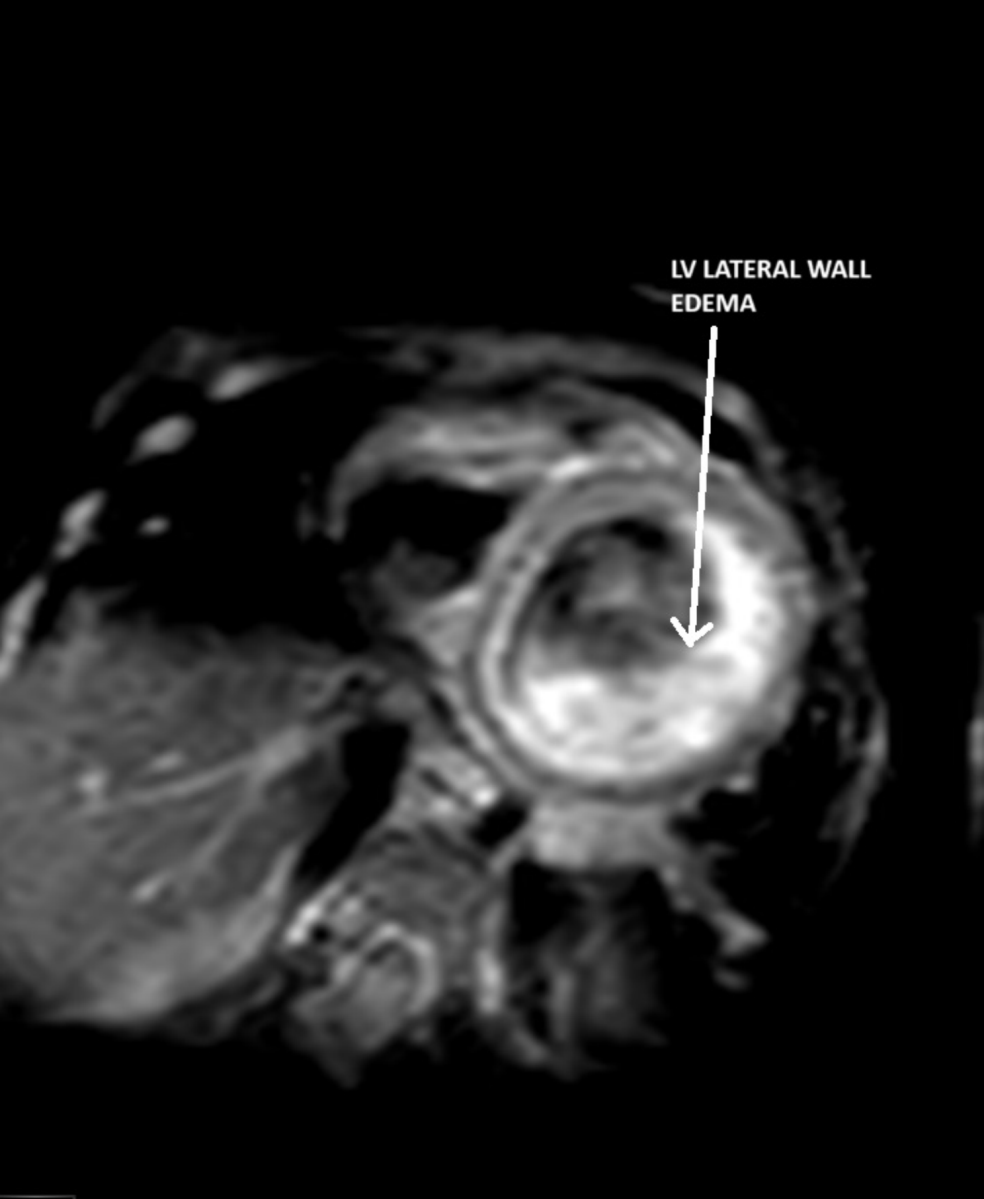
Cardiac MRI scan T2-weighted signal- Lateral LV wall edema
